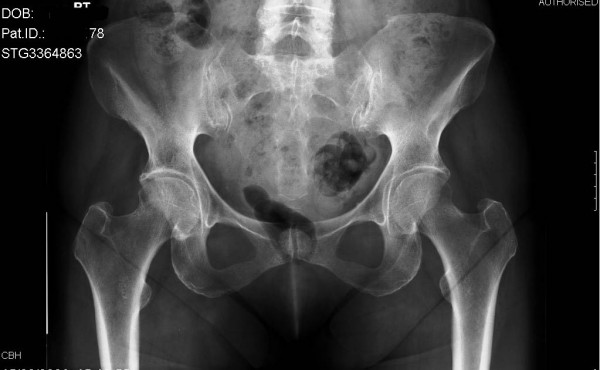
Plain radiograph taken at the time of initial presentation and reported as 'no bony injury'.
